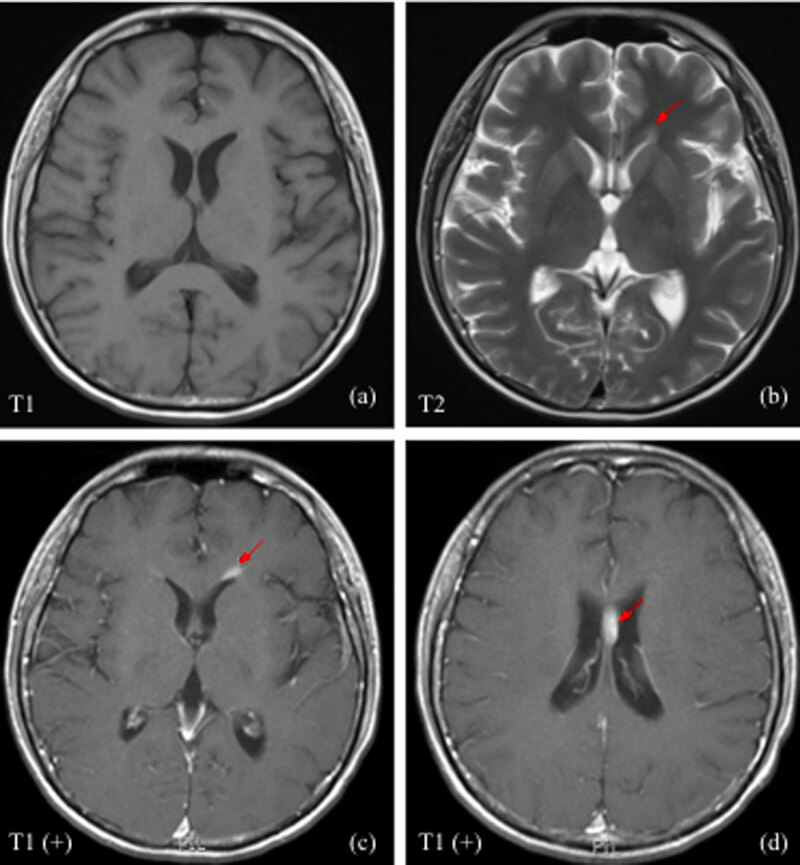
Contrast-enhanced brain MRI: Homogeneous enhancing lesions in the left periventricular region (arrow in c) and septum pellucidum (arrow in d) with isointense on T1WI (a) and hyperintense on T2WI (b).
